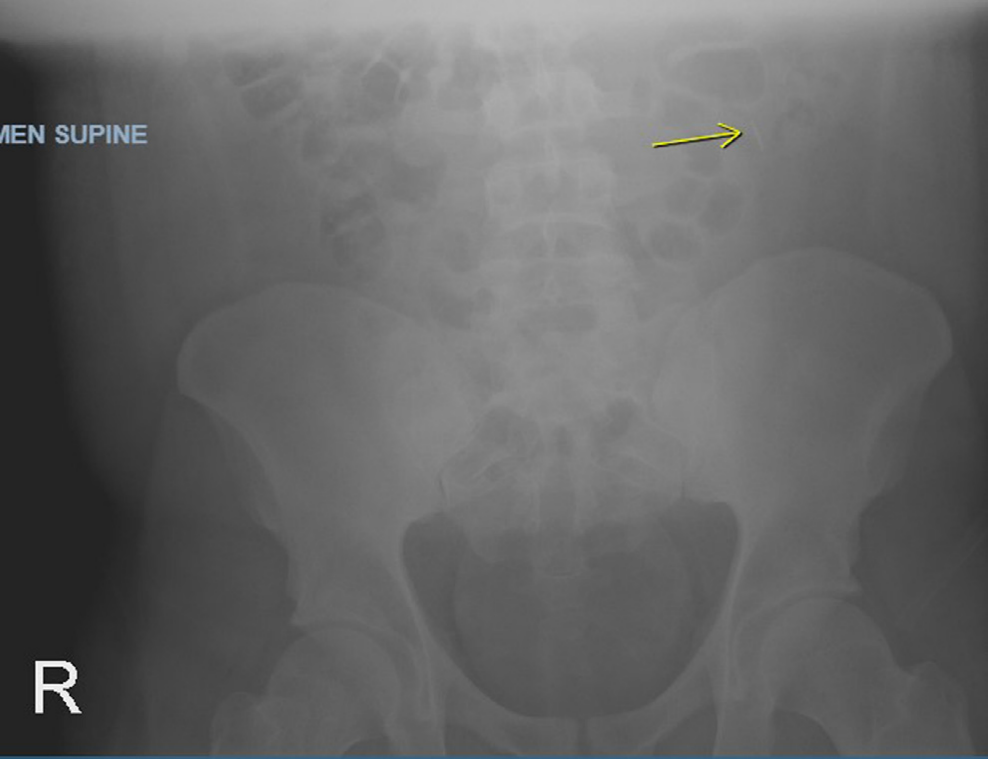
X-ray of abdomen demonstrating foreign object in left mid-abdomen. A repeat X-ray of the kidneys, ureters and bladder revealed a linear radiopaque foreign body overlying bowel loops on the left side of the mid-abdomen (arrow). The exact location could not be identified. No free air noted on film.
